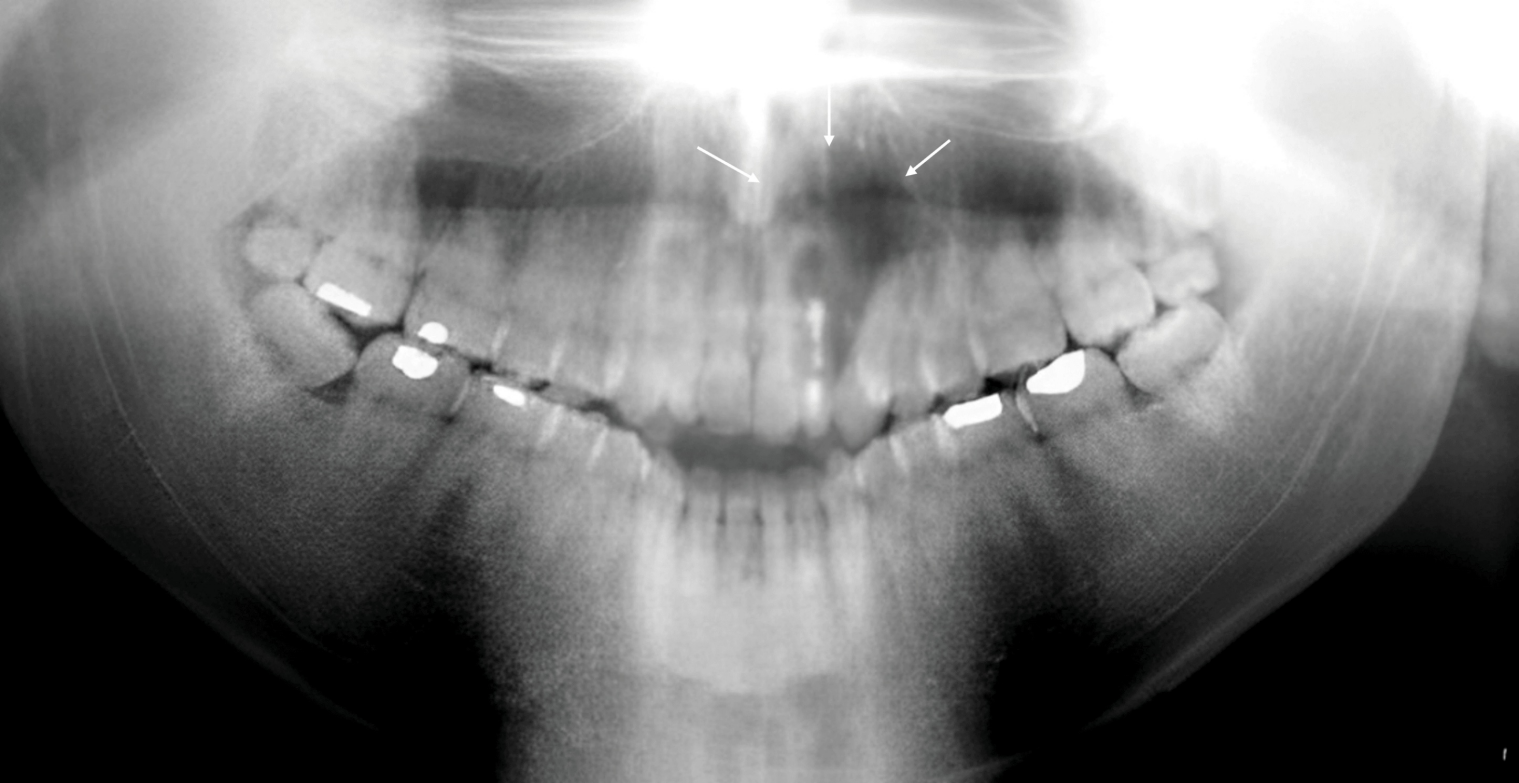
Panoramic radiograph shows a well-defined "inverted pear-shaped" radiolucency, extending from the central incisor to the left first premolar (arrows)
